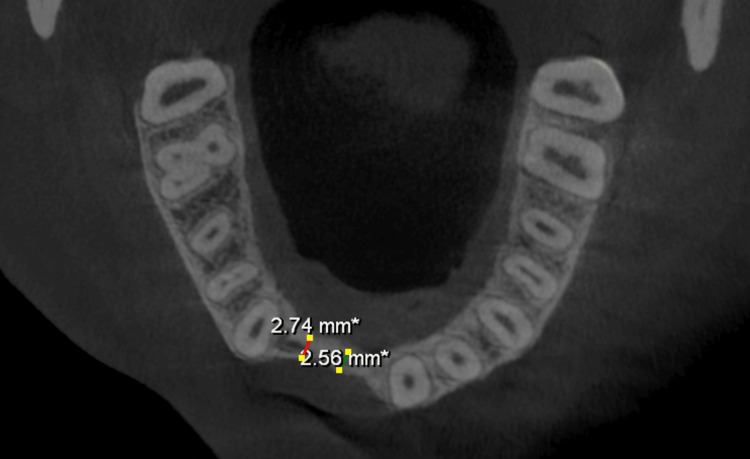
CBCT (axial view) indicating a preoperative maxillary ridge width of 2.56 mm. CBCT, cone-beam computed tomography
